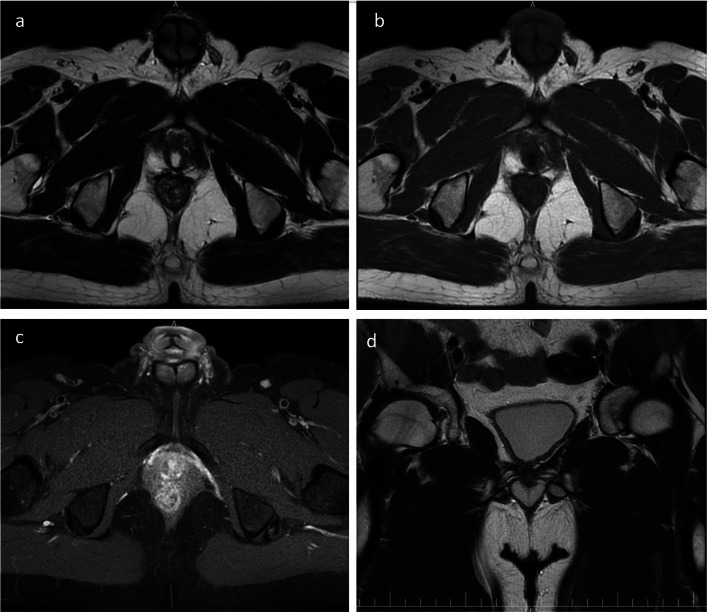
MRI of the prostate: (A) axial T2-weighted, (B) axial T1-weighted FSE, (C) axial T1-weighted with contrast enhancement (CE), and (D) coronal T2-weighted.
